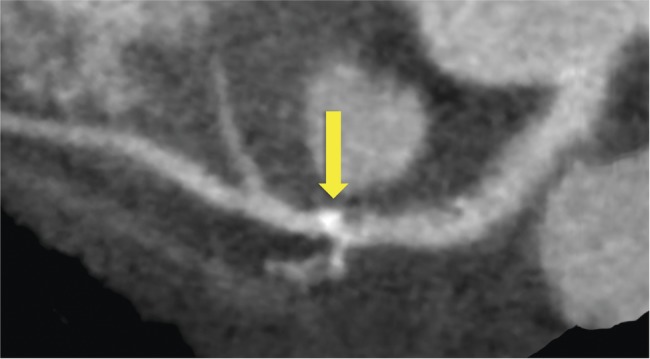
CT coronary angiography curved planar reconstruction of the left anterior descending coronary artery showing an atherosclerotic plaque with calcified and non-calcified components. The location of this plaque, which spans the origin of the first diagonal vessel, can cause differences in segmental classification between observers as it could be classified as proximal left anterior descending artery, mid left anterior descending artery, or both.
